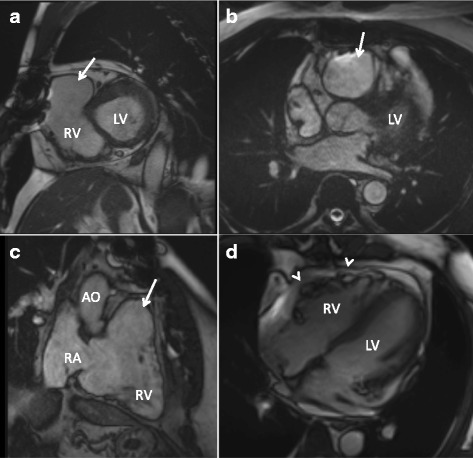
Cardiac magnetic resonance images of a 59-year-old male homozygote for the Thr335Ala in *DSG2* (II.5, family 2). Short-axis (a), axial (b) and right ventricular outflow tract (RVOT) (c) views of the heart show aneurysmal dilatation of the RVOT (arrows). Maximum dimension of the aneurysm was 67 mm and right ventricular end-diastolic volume index (RVEDVI) was 109 ml/m^2^. During follow-up, further dilatation of the aneurysm and the RV was observed. Four-chamber view shows rare microaneurysms in the right ventricular free wall (arrowheads) (d). LV = left ventricle; RV = right ventricle; RVOT = right ventricular outflow tract; RA = right atrium; AO = aorta
